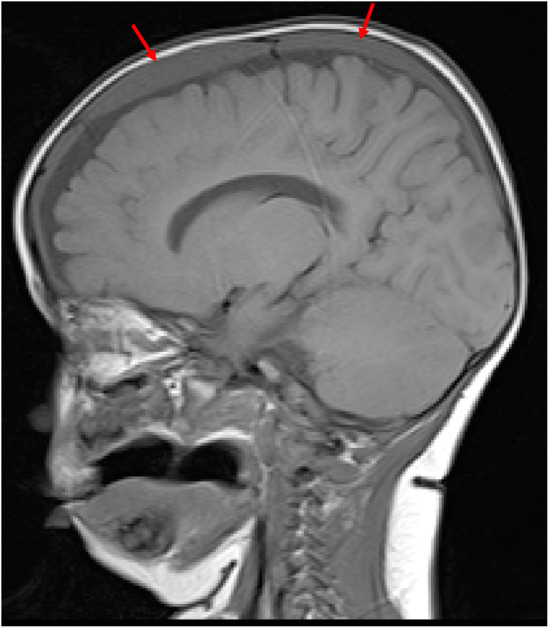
T1 weighted sagittal MRI 7 days after the fall injury. There is a holohemispheric isointense subdural collection present on the left. There is a smaller collection on the right (not shown).
